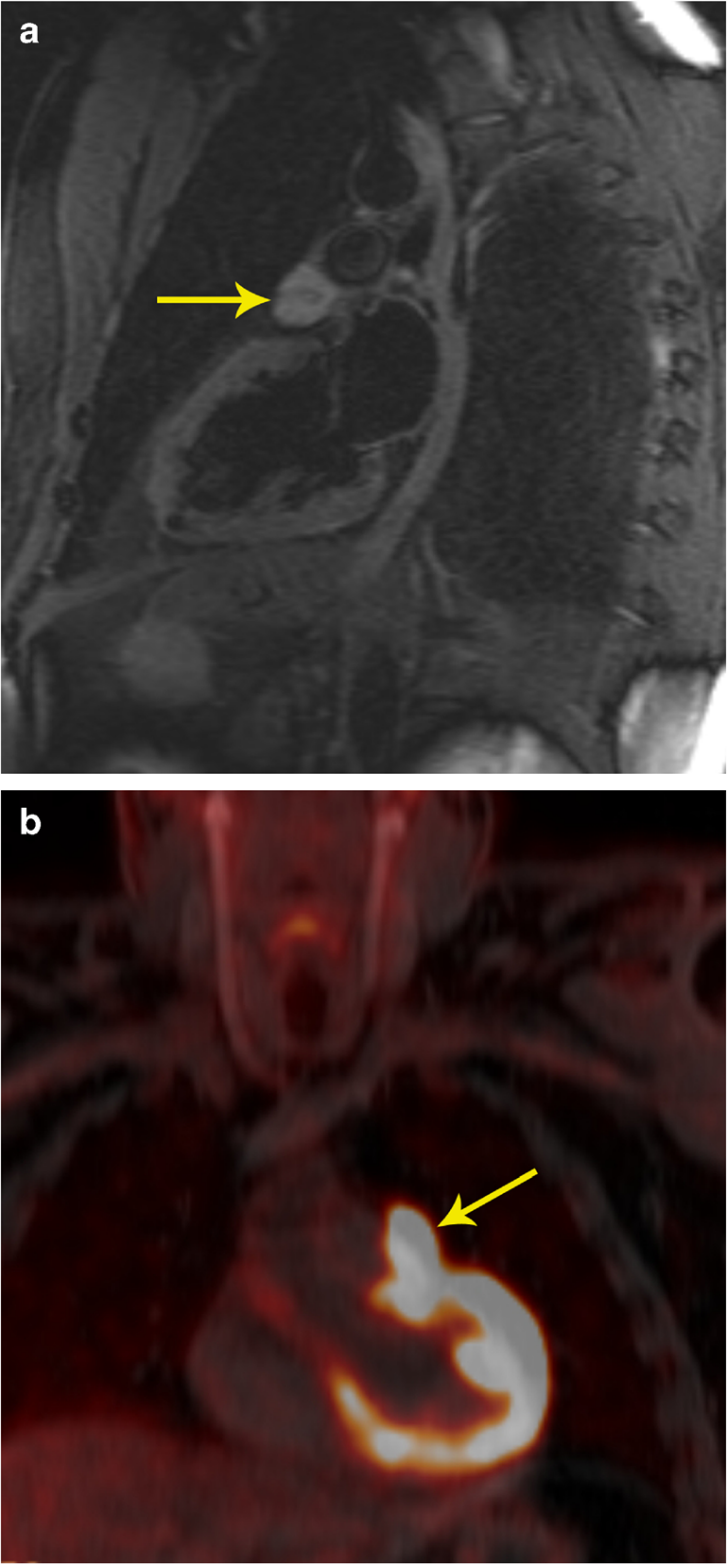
Characterization and staging. a 2-chamber STIR image obtained as a part of FDG-PET/MRI examination in a patient with cardiac mass shows a well-defined hyperintense mass adjacent to the left atrial appendage (arrow). This mass also showed intense contrast enhancement (not shown here). b The fused FDG- PET/MR image in the coronal plane shows intense uptake in the mass (arrow) adjacent to the left ventricle. This was shown to be a paraganglioma. The FDG-PETT scan also ruled out any other metastatic lesions, indicating that the lesion was benign.
